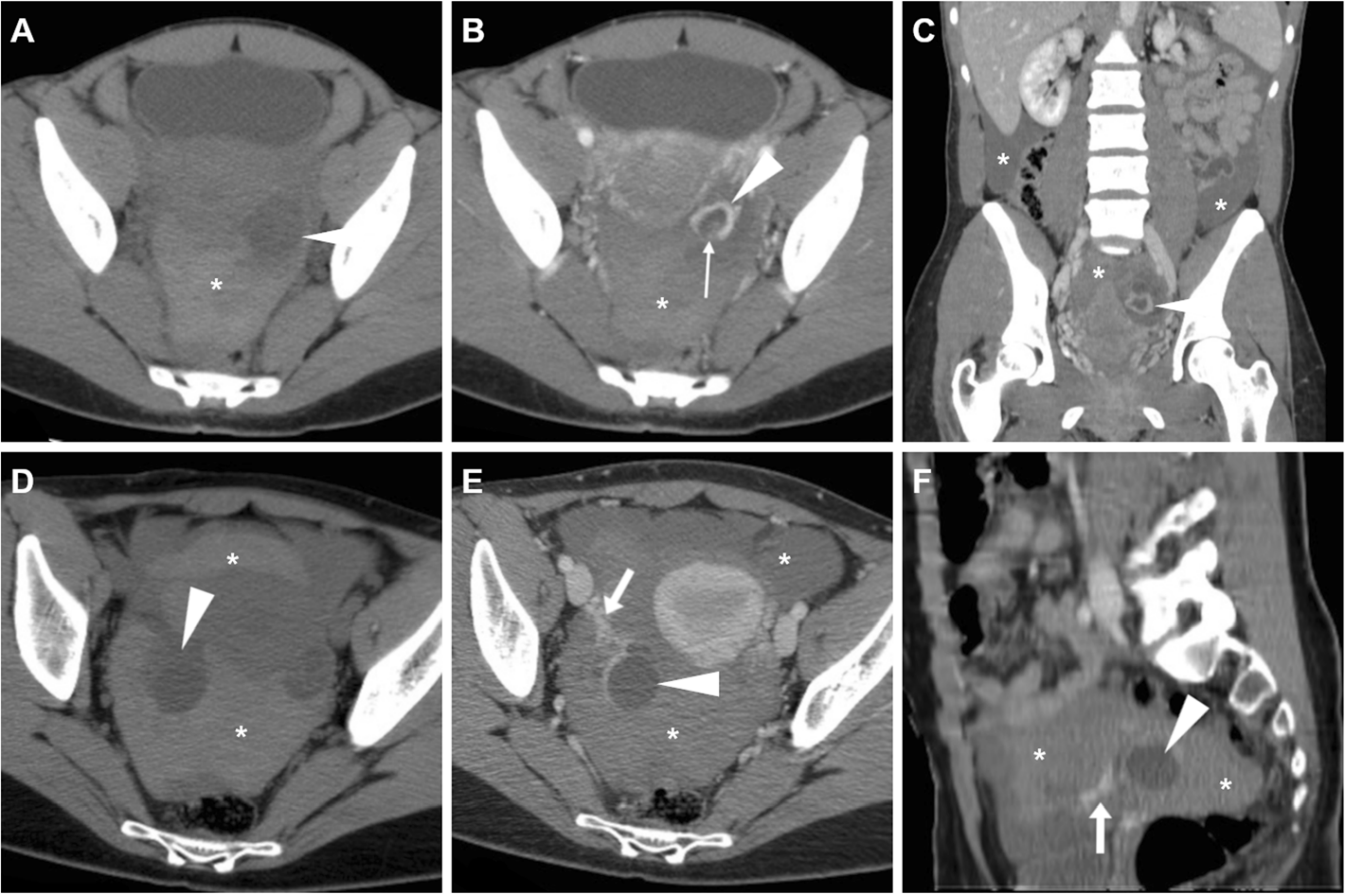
Two cases of haemoperitoneum from bleeding corpus luteum. a–c Unenhanced (a) and post-contrast (b, c) CT show hyperattenuating effusion (asterisk) in pelvis and paracolic gutters, mildly enlarged left ovary containing a characteristic corpus luteum (arrowheads) with focal discontinuity (thin arrow in b) of its strongly enhancing crenulated wall. d–f Unenhanced (d) and post-contrast (e, f) CT images show pelvic blood (asterisk) surrounding a 3-cm cystic lesion of the right ovary (arrowheads), from which contrast extravasated (arrows in e and f) indicating active bleeding
